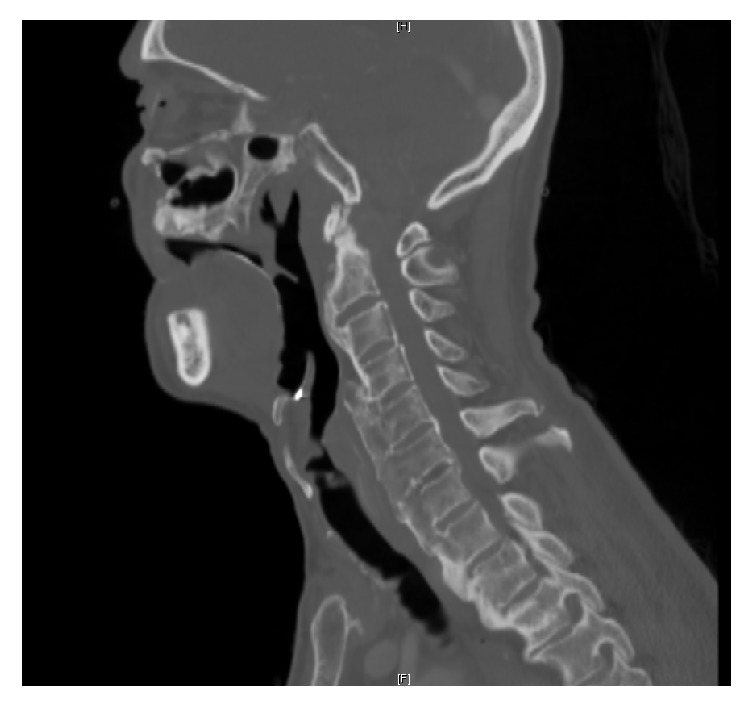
CT scan of the cervical spine reveals continuous and irregular hyperostosis alongside the anterior aspect of the cervical spine consistent with DISH.
